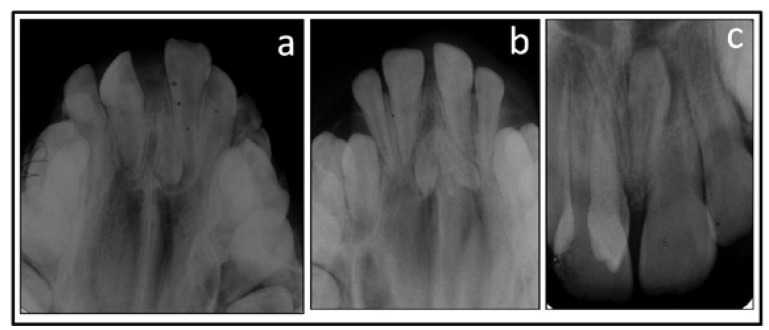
Occlusal radiograph showed one (a) and two (b) inverted supernumerary teeth at the midline, one inverted and one normal position supernumerary tooth (c).
